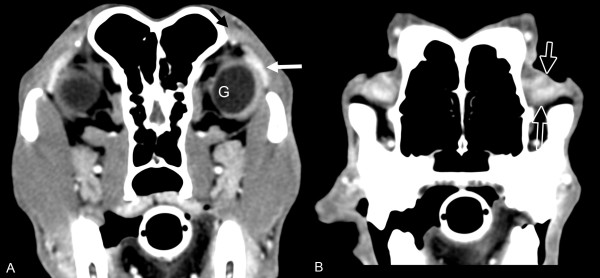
Transverse contrast-enhanced CT images of the lacrimal glands. Image markers A-B denote the right side of the image. (A) The lacrimal gland (white arrow) and (B) accessory lacrimal gland of the third eyelid (open arrows) are markedly contrast-enhancing structures on postcontrast 1.25 mm CT images.
